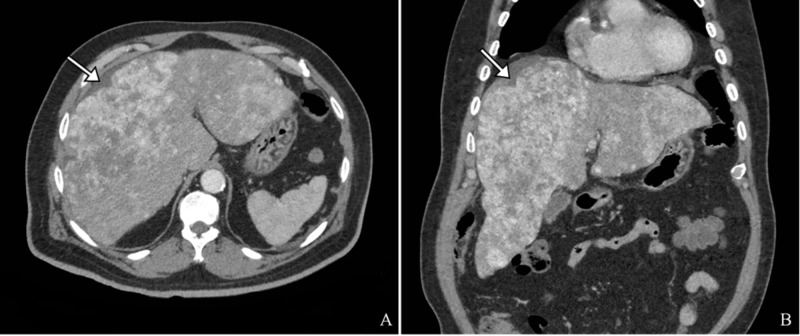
Representative CT images Contrast-enhanced CT images in the axial (A) and coronal (B) planes demonstrate a diffusely enhancing, multifocal, heterogeneous, and infiltrative mass occupying most of the hepatic parenchyma. Mild capsular retraction (arrows) is noted secondary to the mass at the periphery of the liver. CT findings are highly concerning for a primary or metastatic hepatic malignancy.
